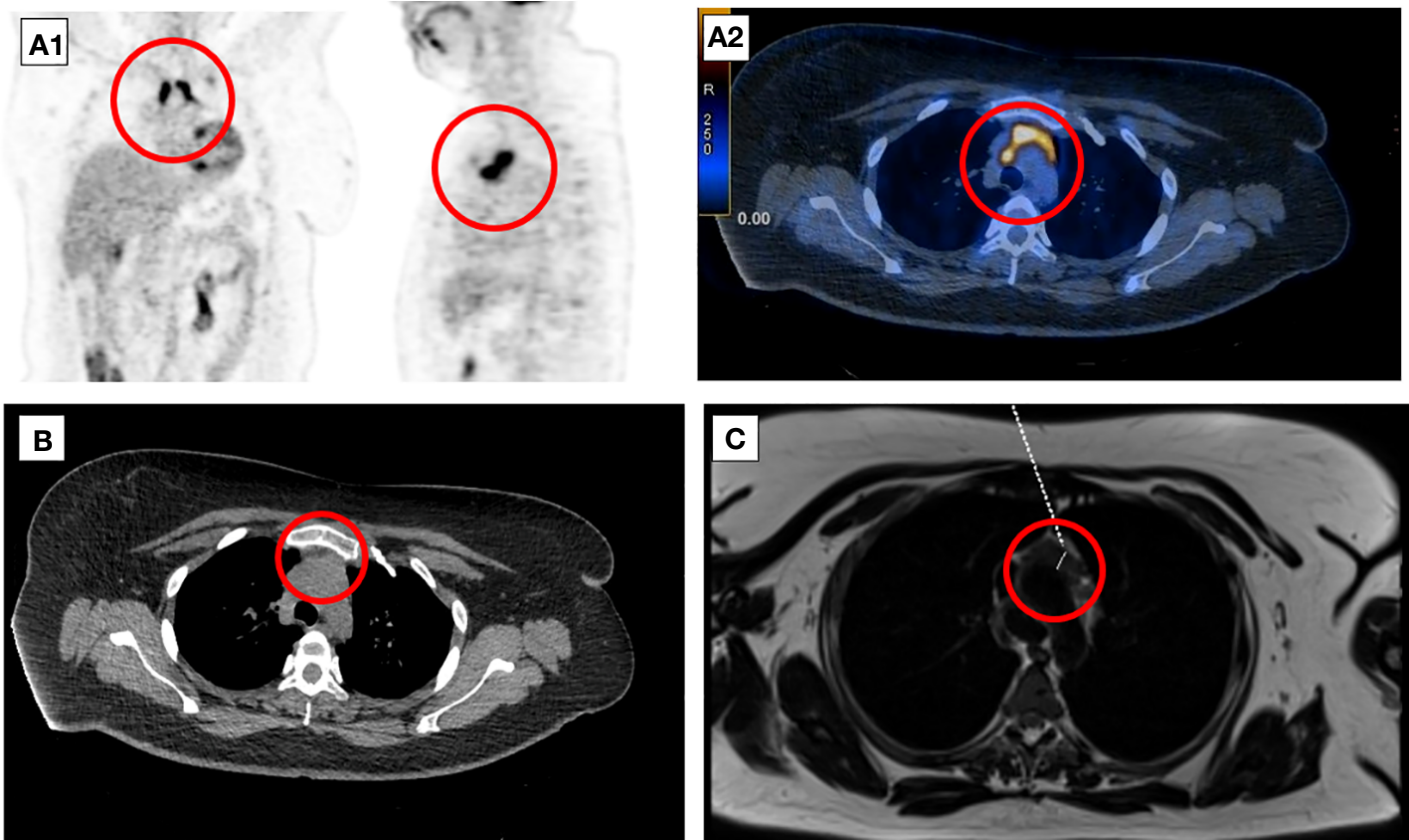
Eight-week follow-up 18F-FDG-PET-CT presenting metabolically active lesion in the superior anterior mediastinum in the thymus location (A1, A2). Axial CT (B) and MRI (C) scans of the chest presenting mediastinal mass corresponding to the invasive thymic malignancy.
